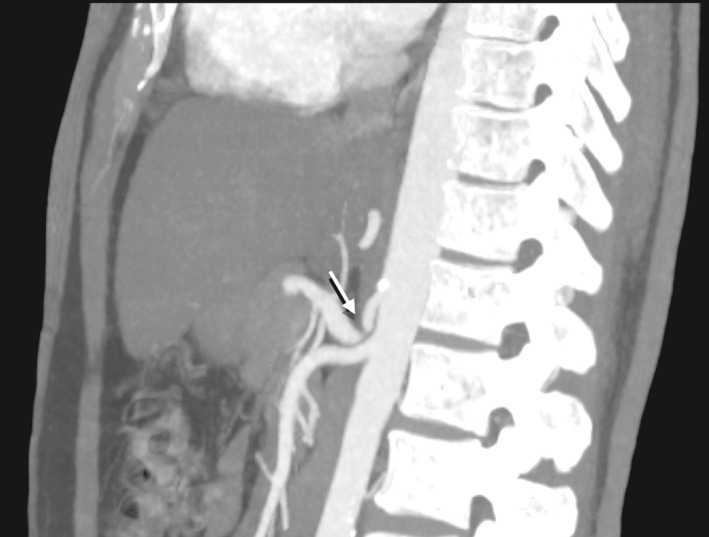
Computed tomography angiography (CTA) of the abdomen of the patient, parasagittal reconstruction showing a narrowed celiac artery with hook‐like configuration *(white arrow)* due to indentation of median arcuate ligament in the superior aspect of the celiac artery consistent with median arcuate ligament compression. Mild poststenotic dilatation of celiac artery can be visualized
